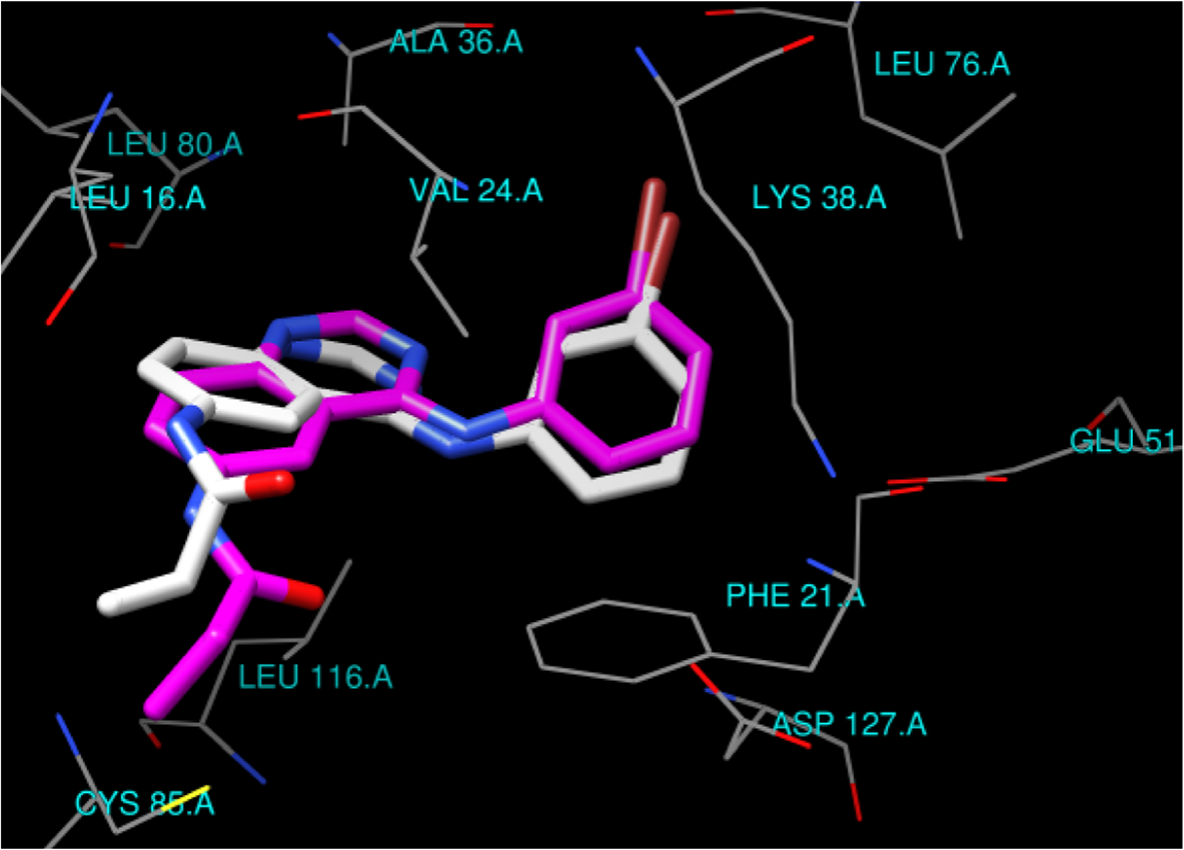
N*-[4-(3-bromophenylamino)quinazolin-6-yl]acrylamide (DJK_3021_A) from 2J5F (X-ray magenta, docked white) oriented epidermal growth factor receptor (EGFR) kinase binding site.
